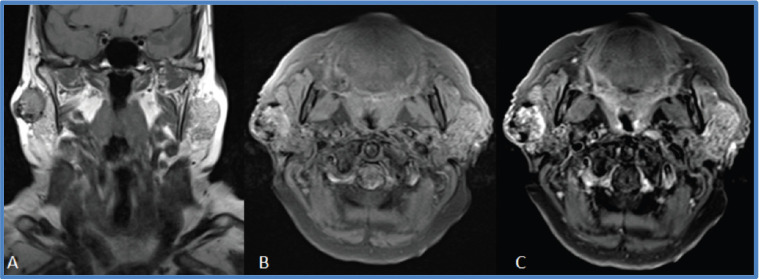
Coronal MRI section in T1 sequence. (a). Right superficial parotid nodule with a heterogeneous signal, well-defined edges and an apparently fibrous capsule. (b and c): Axial MRI section in T1 FAT SAT sequence with and without gadolinium. This nodule shows a predominantly peripheral enhancement after gadolinium administration.
